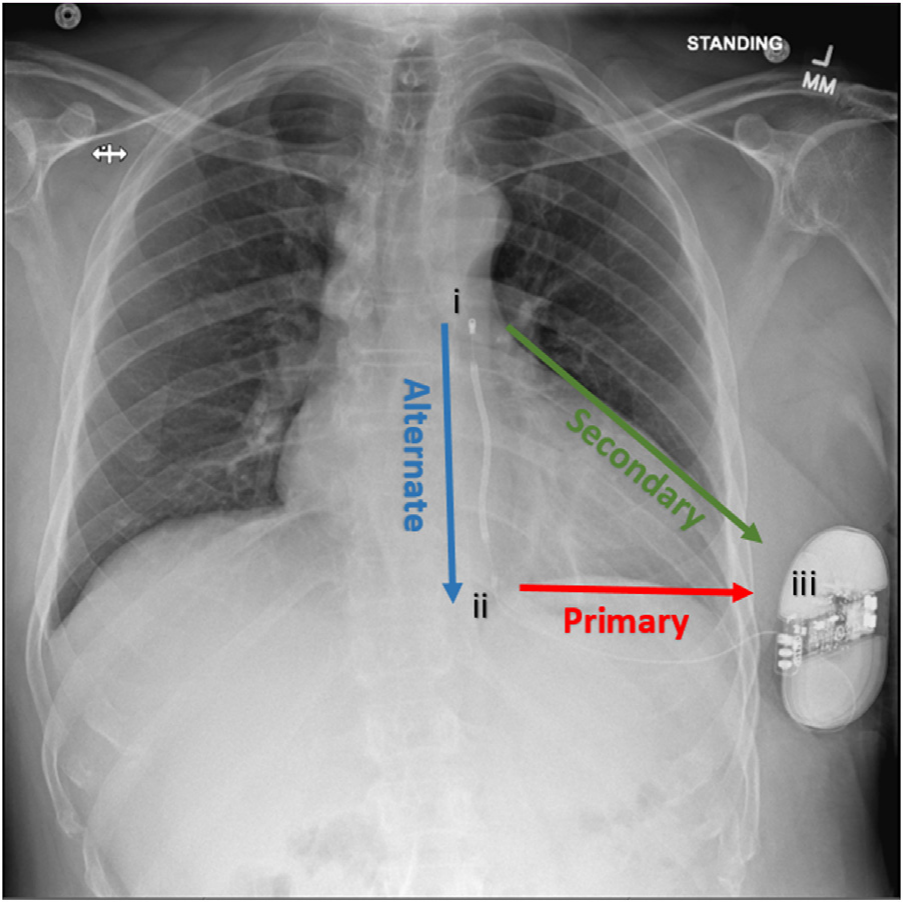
Anteroposterior chest radiograph with subcutaneous implantable cardioverter-defibrillator position and sensing vectors. The 3 available sensing vectors are shown: Primary vector is from proximal electrode ring to can; Secondary vector is from distal electrode ring to can; and Alternate vector is from distal to proximal electrode rings (i represents the distal electrode ring, ii represents the proximal electrode ring, iii represents the pulse generator).
